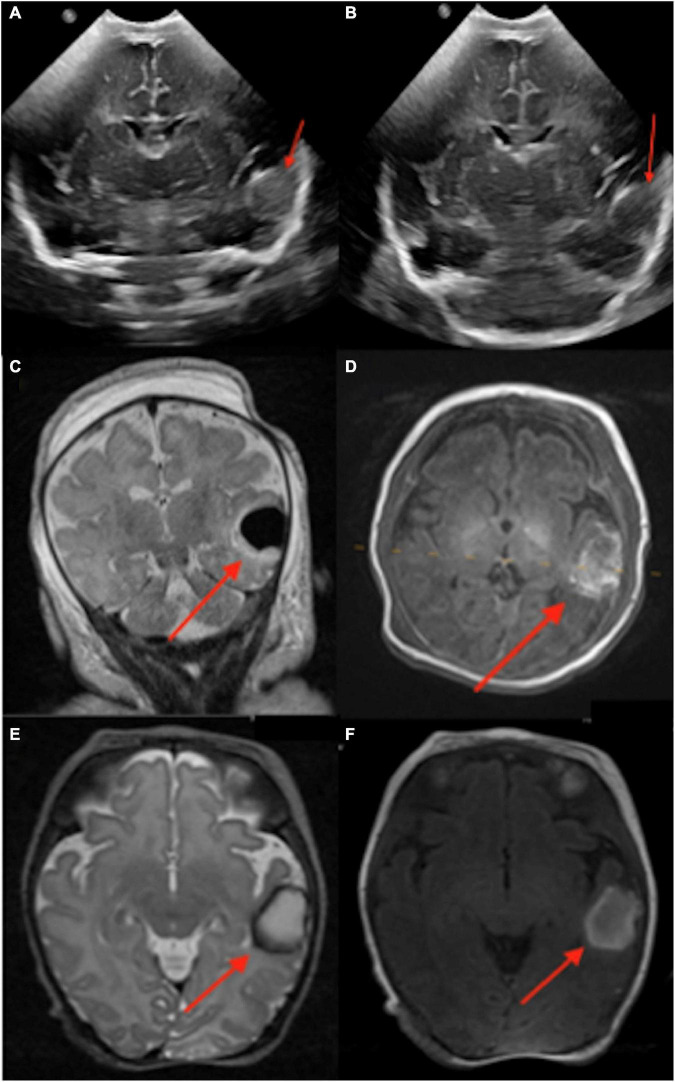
An infant born at 37 weeks and 3 days, with delivery complicated by shoulder dystocia and brachial plexus injury, was found to have hypoglycemia and seizures after birth. A left temporal lobe intraparenchymal hemorrhage was found in transcranial ultrasound (1 day old, A,B). This was further delineated on conventional 3-Tesla MRI (4 days old, C,D) and followed on point-of-care 1-Tesla MRI (35 days old, E,F).
